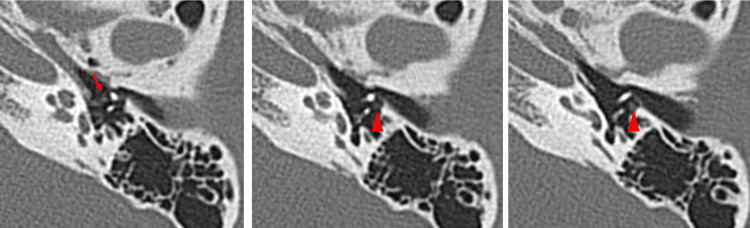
Three consecutive sections (0.5 mm thickness) of axial HRCT images of the left temporal bone (Case 2) showing an abnormal soft tissue lesion appearing at the medial side of the malleus (arrow) and at the lateral side of the stapes (arrowheads). HRCT, High-resolution computed tomography
